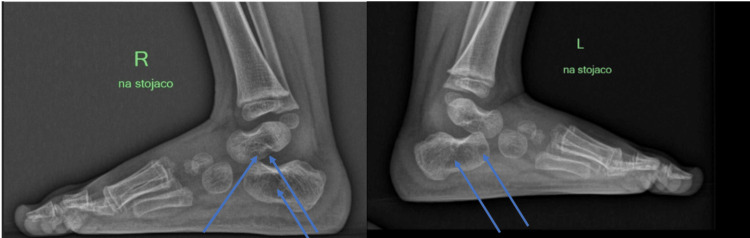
Lateral X-rays of the feet before treatment The arrows indicate poor bone density with bone cysts and cavitation
